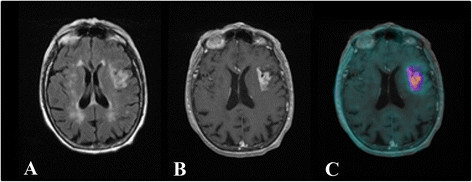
Axial images obtained after vascular injury. The imaging studies were performed 13 days post-stroke. The infarct volume evaluated on MRI was 13 cm^3^. (A) Flair T2-weighted MR image. Left fronto-insular hypersignal demonstrating infarcted tissue. (B) Post-gadolinium T1-weighted MR image. Enhancement signal in the left fronto-insular cortex corresponding to the breakdown of BBB. (C) Co-registration image obtained between the post-gadolinium T1 MRI and PET. Note the absence of ^18^ F-DPA-714 uptake by the multiple bilateral nodular hypersignals within the white matter in connection with leukoaraïosis lesions.
